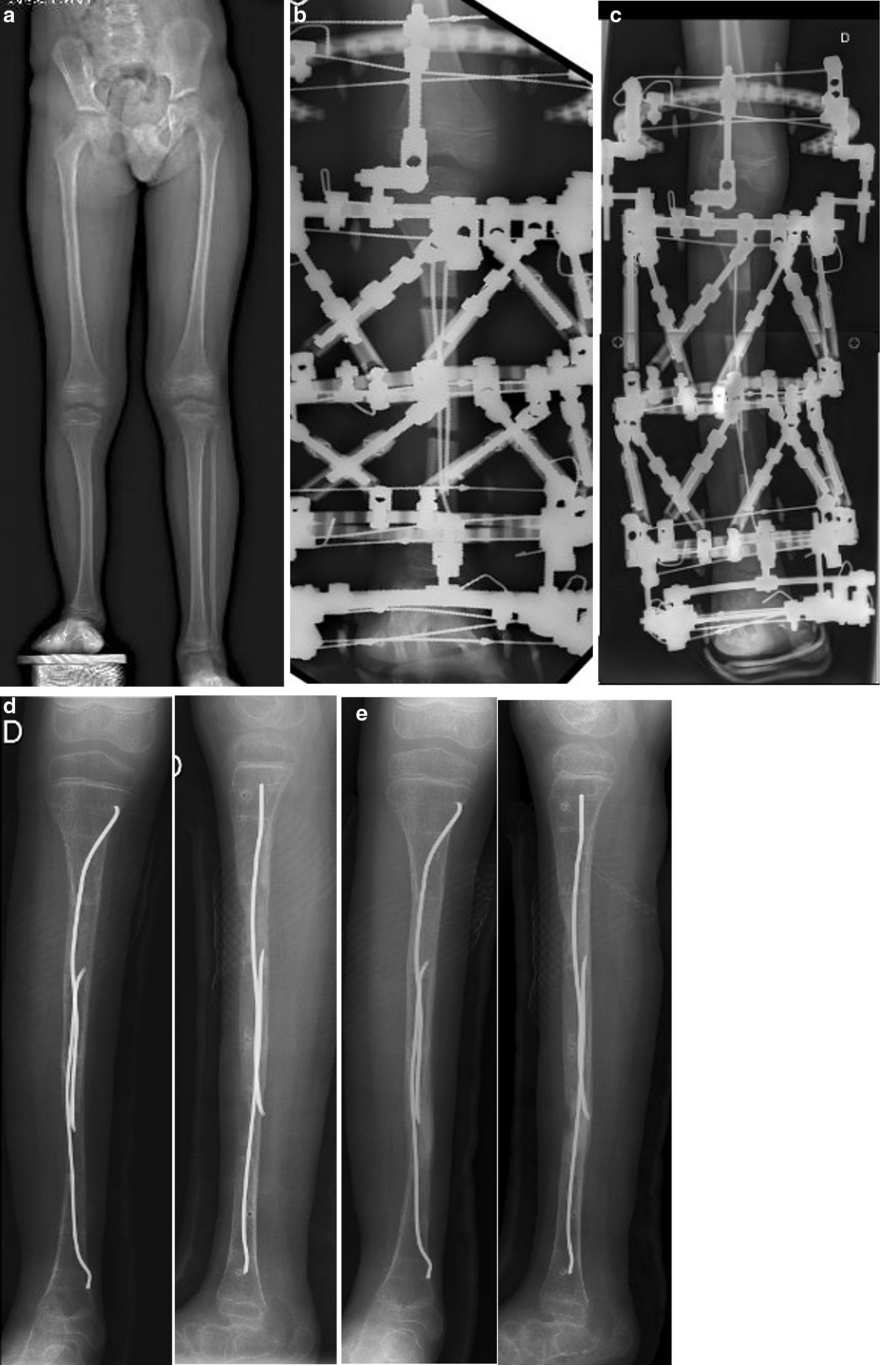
Bipolar tibia lengthening in patient with fibular aplasia: a X-ray image of lower limbs before treatment; b day 10 of distraction period; c day 46 of distraction period (end of lengthening); d day of frame removal, distal regenerated bone is weak, without anterior cortex, but protected by intramedullary nail; e 6 weeks after frame removal, distal regenerated area is solid, with thickened cortices and no angular deformity
